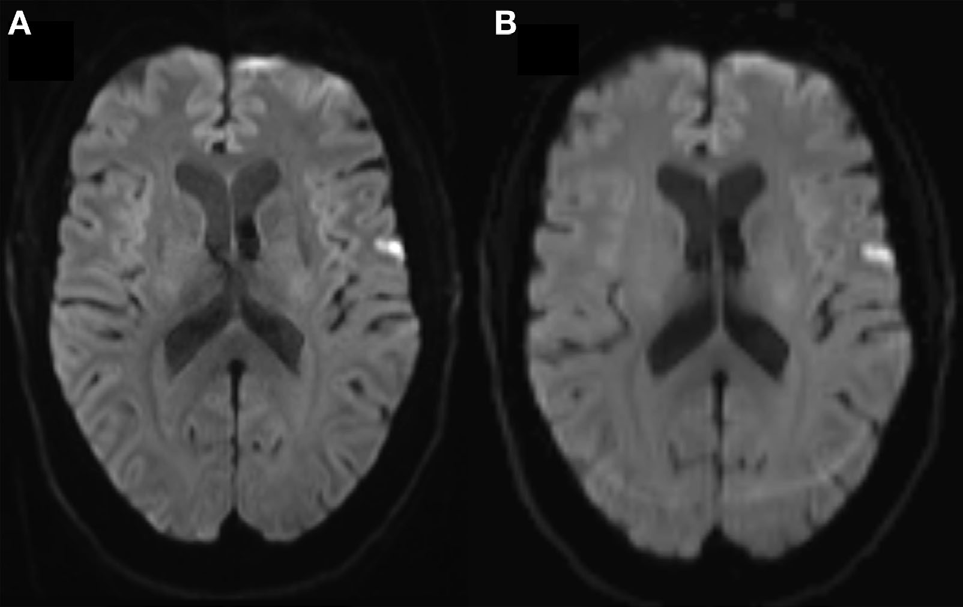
(A) Three and (B) 20-gradient direction diffusion-weighted imaging (DWI) images of 68 years female with 2 h lasting transient ischemic attack symptoms including right hand paresis and slurred speech. In (B), note the occipital ring artifact, blurred contours of the right-sided cortical diffusion lesion and blurred cortex outline due to motion. Three-gradient direction DWI had acquisition time 2 min and 7 s and 20-gradient direction DWI had 4 min and 39 s, both were standard vendor protocols.
